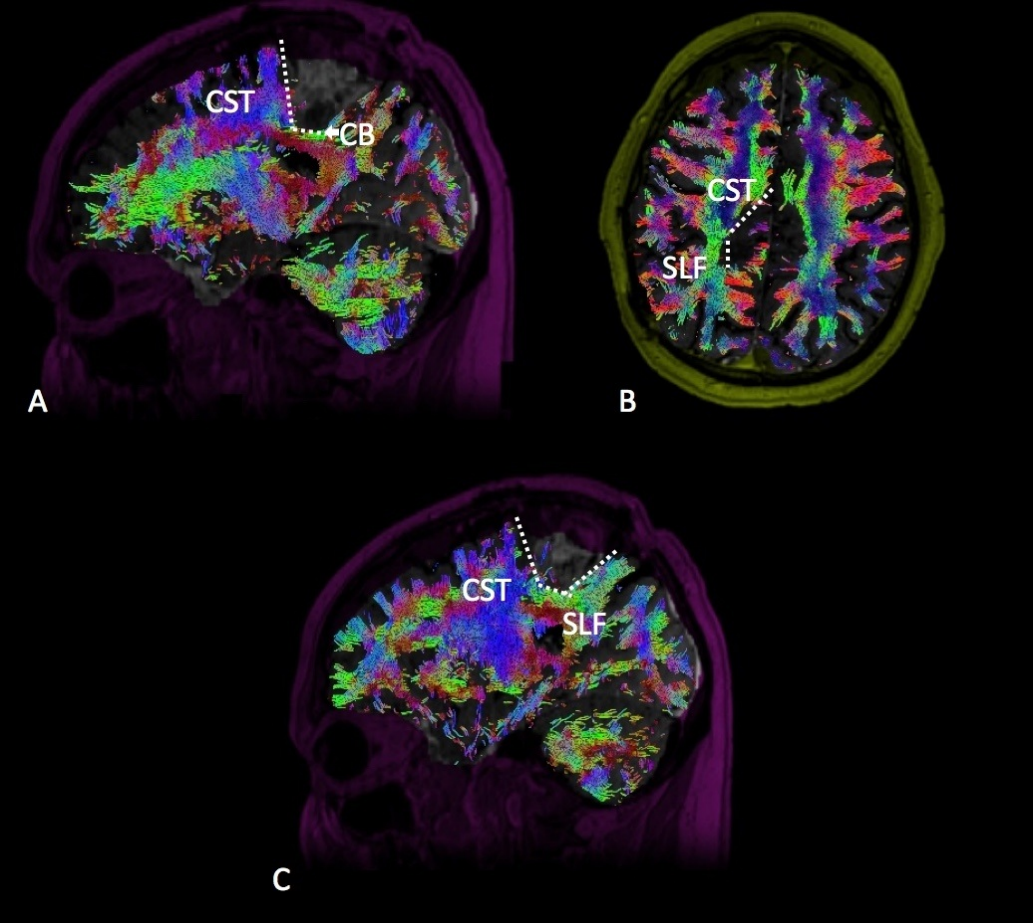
Medial Parietal Diffusion tractography enhanced T1 magnetic resonance imaging (MRI) with contrast with (A) sagittal, (B) axial, and (C) sagittal views demonstrating a medial parietal glioma in relation to the surrounding white matter tracts. Labeled white matter tracts associated are shown as follows: superior longitudinal fasciculus (SLF), corticospinal tract (CST), and cingulate bundle (CB). The disconnection pattern is shown as a white dotted line.
